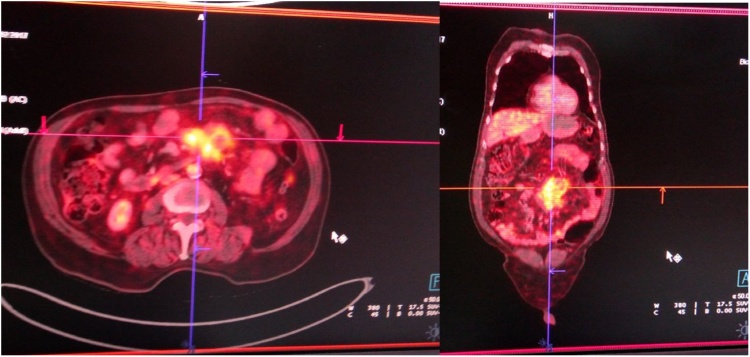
PET/CT scan showing FDG avid mass in the mesentery.
